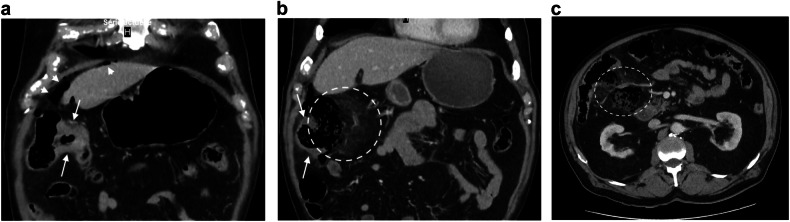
Stercoral peritonitis due to colonic cancer with upstream perforation. Coronal and axial portal phase CT (a–c) in a 78-year-old man shows free intra-peritoneal gas (arrowheads) and feces associated with fat stranding (dotted circle). The site of the perforation is located above a short-segment thickening of the right colonic wall (arrows), showing a perforation due to distension above a tumoral stenosis
